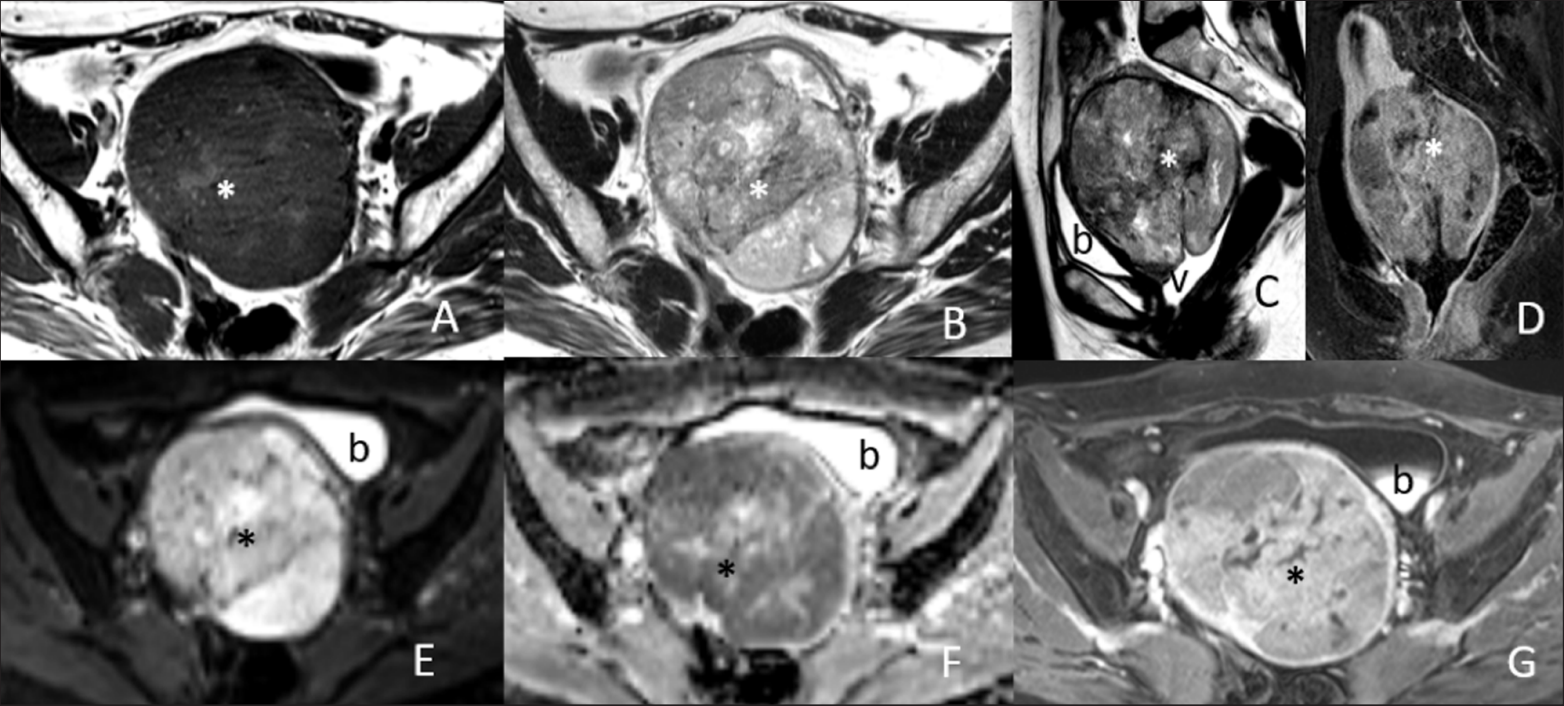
65-year-old woman with LMS. (A) Axial T1WI, (B) axial T2WI, (C) axial diffusion and (D) ADC maps MRI show a large, heterogeneously predominantly T1 low signal and T2 high signal LM (asterisk). Bladder (b).
